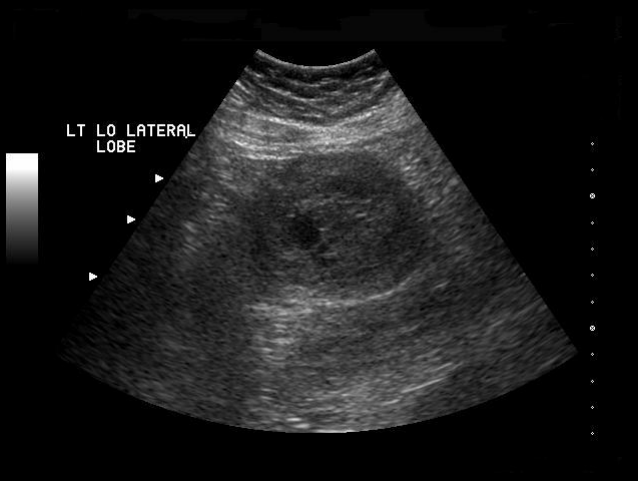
Ultrasound of the abdomen; Ultrasound of the abdomen depicting a 6.3 × 5.3 × 5.0 heterogenous solid mass in the lateral segment of the left lobe of the liver.
